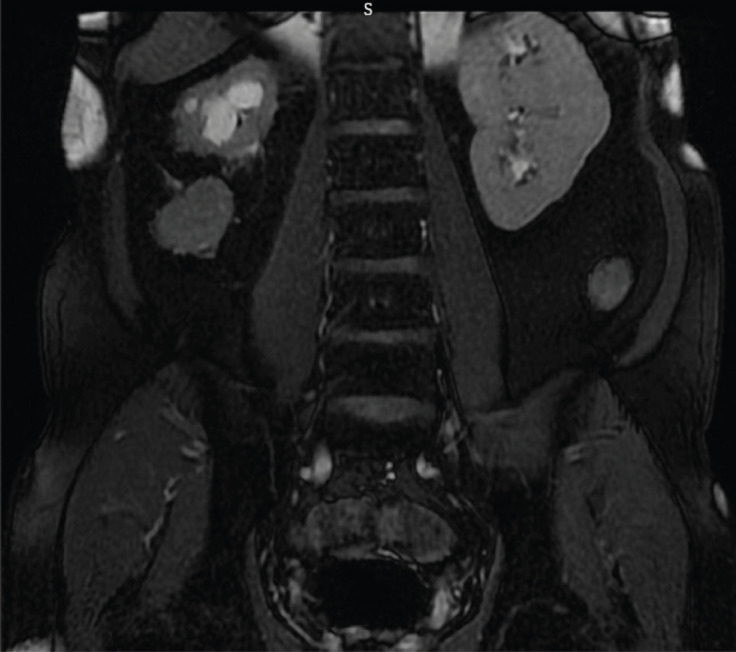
Coronal T2 image of MRI abdomen reveals the tract around right kidney.
